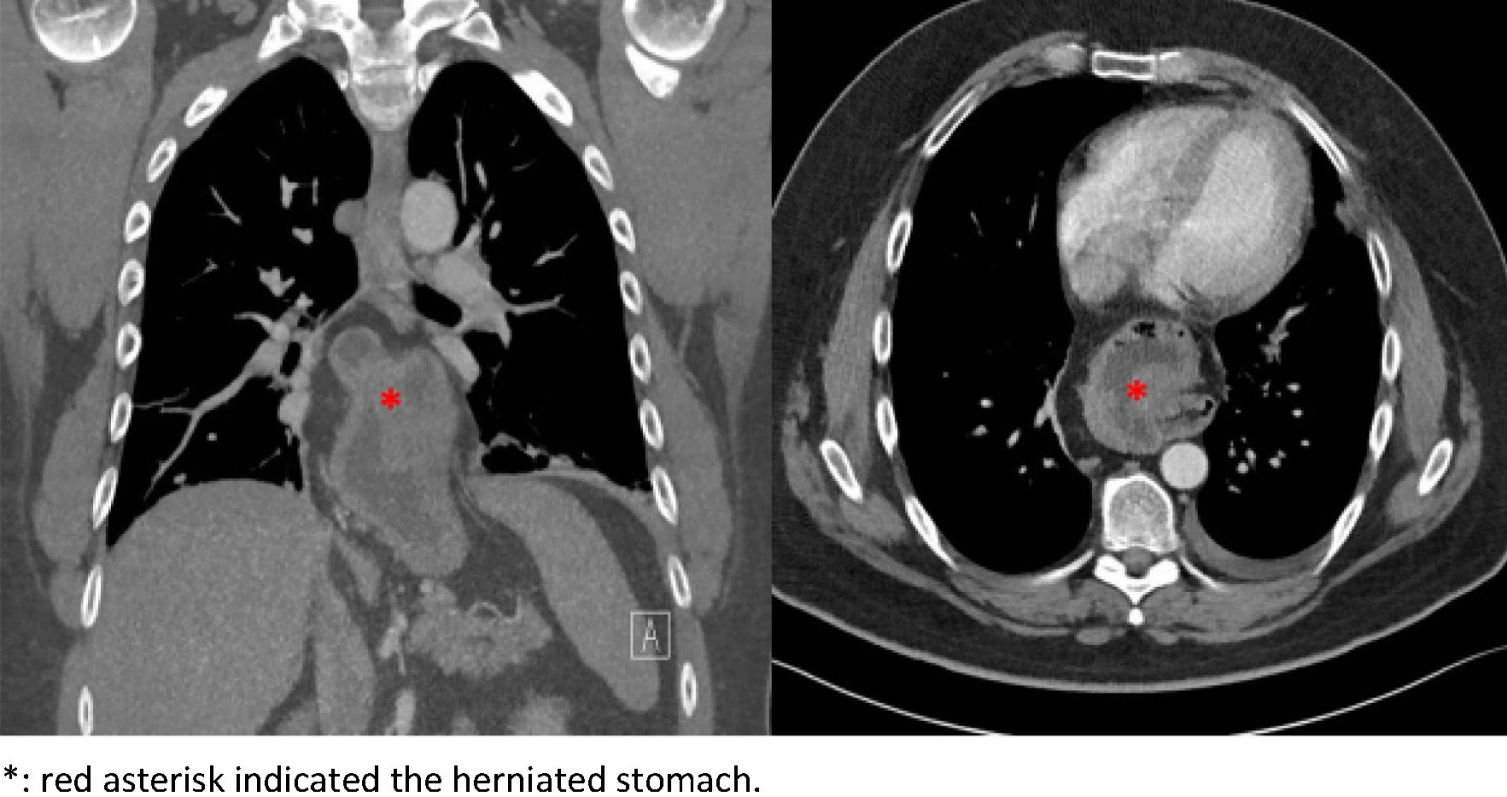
Exemplary CT scan of a patient with upside-down stomach and a BMI of 40 kg/m^2^
